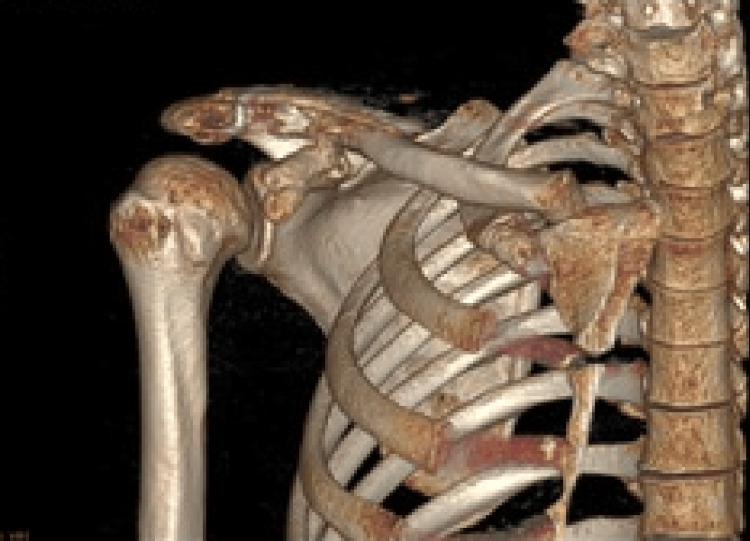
CT 3D reconstruction demonstrating a displaced Ogawa type I basal coracoid fracture
